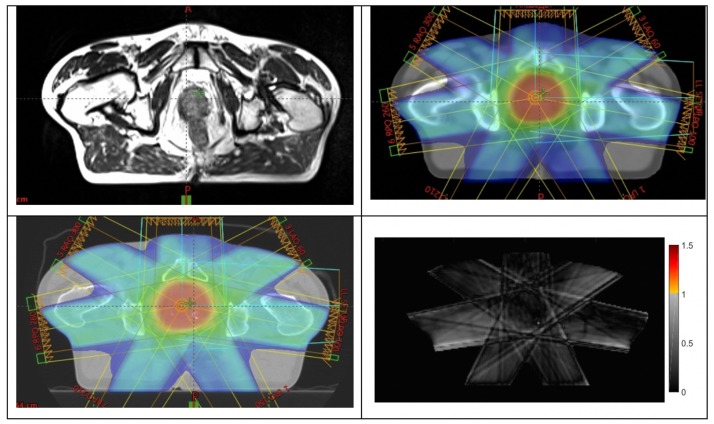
Example of a patient (top-left) large-field-of-view MRI scan; (top-right) dose plan developed on pseudo-CT; (bottom-left) dose recalculated on QA-CT scan; (bottom-right) gamma analysis result at 2%, 2 mm criteria.
